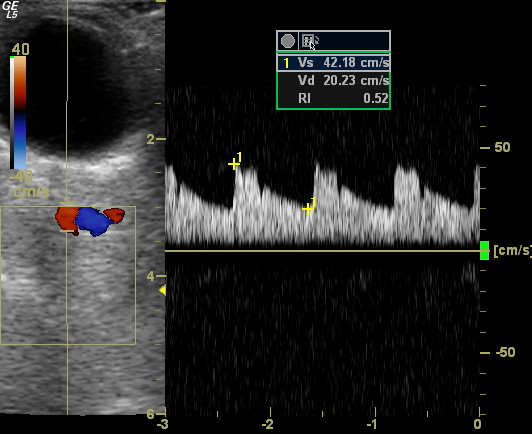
Assessment of ophthalmic artery resistance index with patient lying supine with eyes closed and using an ultrasound frequency of 7.5 mHz. The transducer is applied to the closed upper eyelid using a thick layer of acoustic gel, minimizing pressure on the globe.
